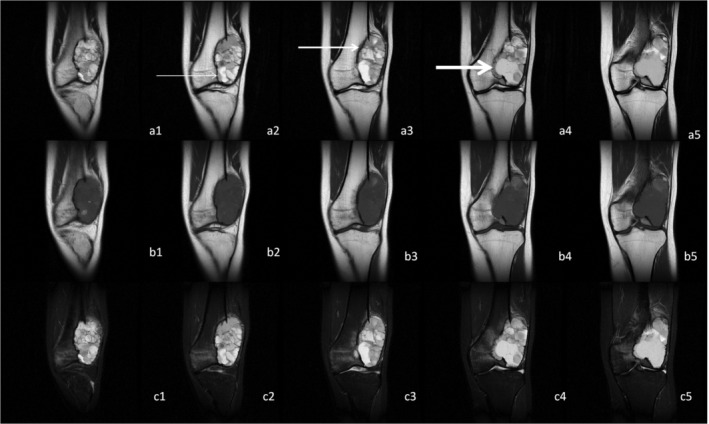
Giant tumor of bone (GCTB) located at distal femur. a1‐a5, b1‐b5, and c1‐c5 were continuous T1WI, T2WI, and T2WI/FS, respectively. The thin arrows, thicker arrows, and thick arrows refer to clear boundary, clearer boundary, and blurred boundary of GCTB, respectively. The boundaries were incomplete with continuous observation of MRI.
